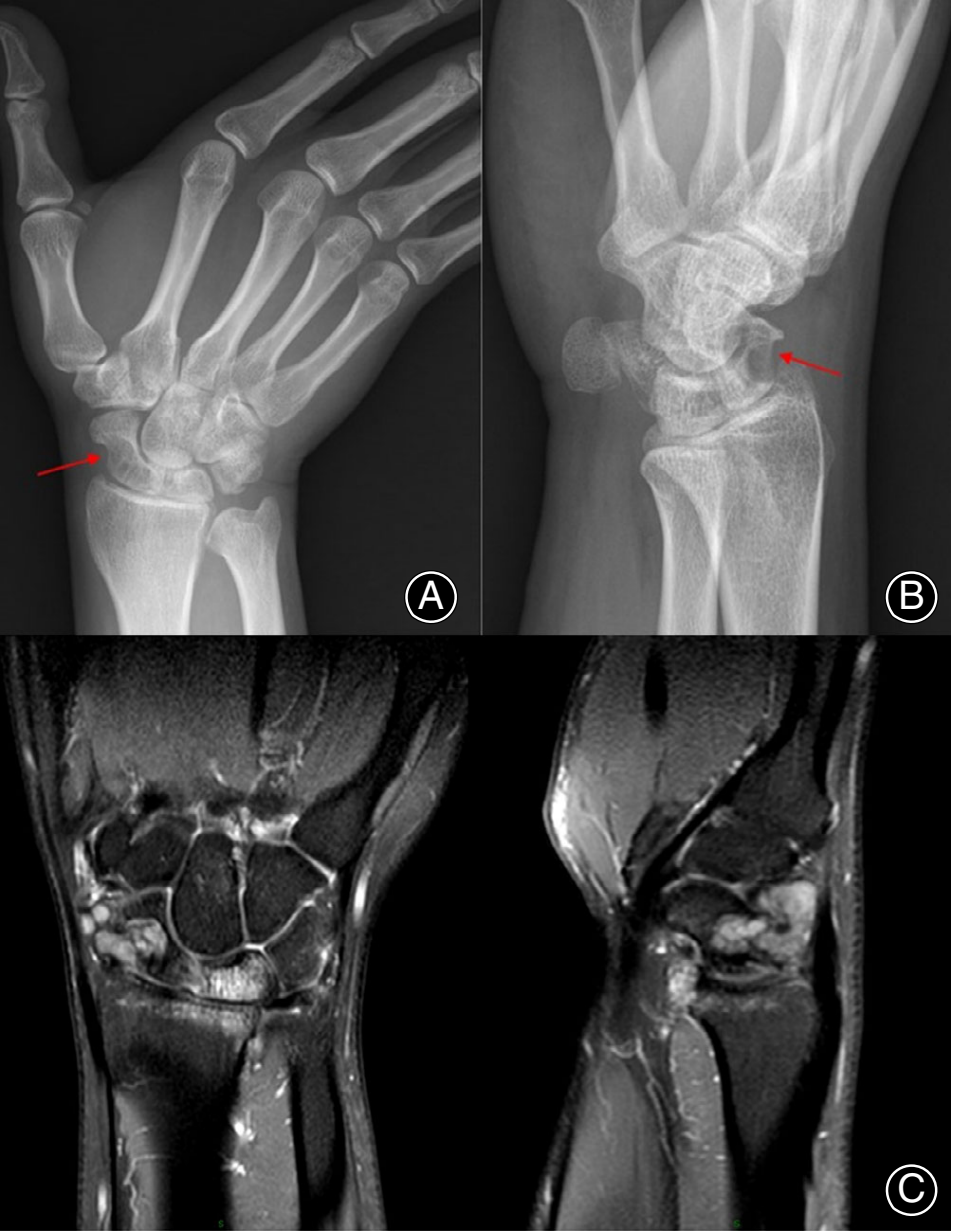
(A. B) X‐rays of the right wrist showing scaphoid deformity with radial aspect concavity suggestive of a local compressive effect (red arrows). (C) MRI of the right wrist showing the infiltrative bony tumor of the scaphoid.
